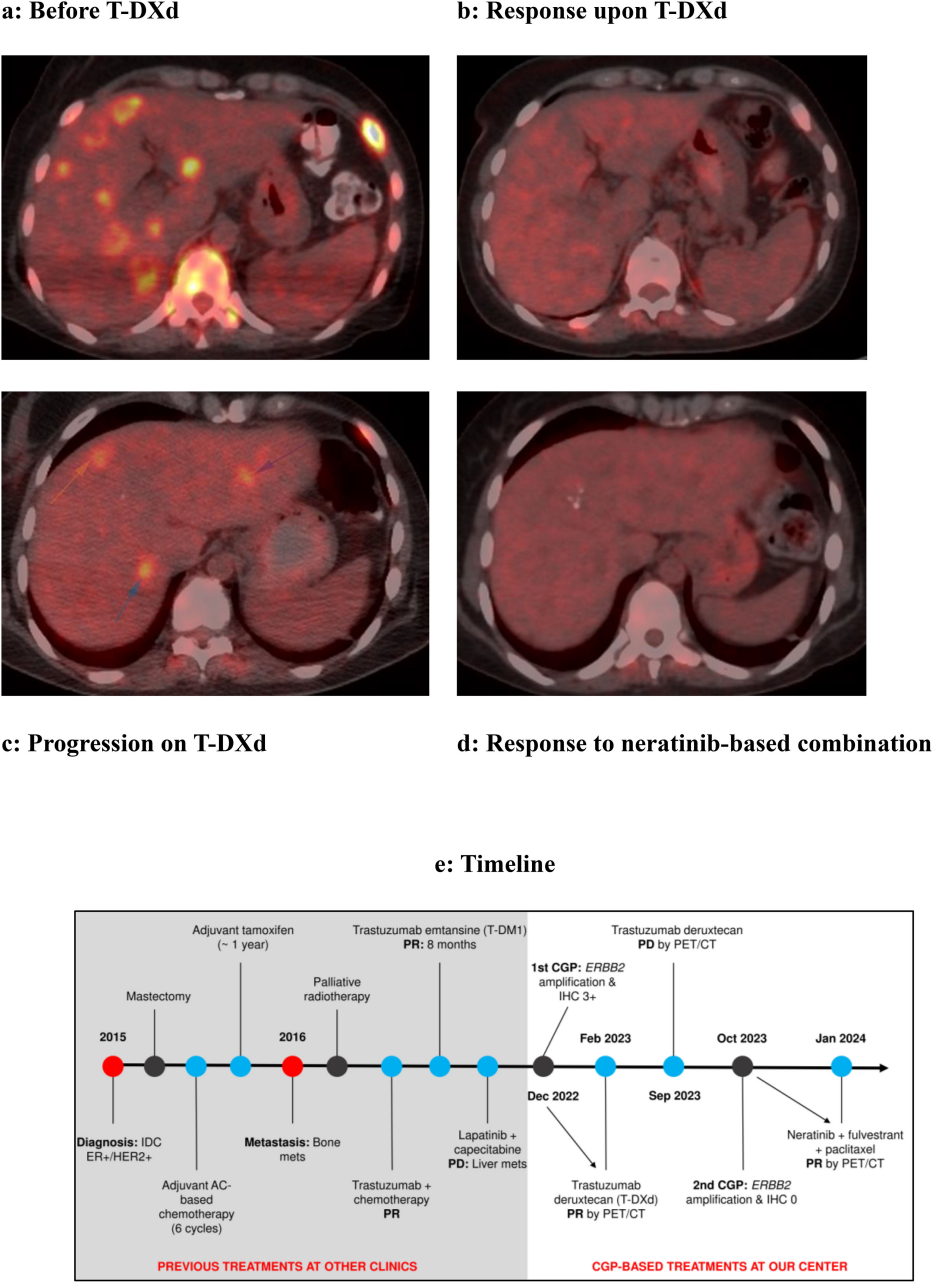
Near-complete responses in an HR+/*HER2-*amplified metastatic breast cancer patient, initially with trastuzumab deruxtecan (HER2 IHC–positive) and then with a neratinib-based combination (IHC-negative). (A) The December 2022 PET result showed multiple lesions with FDG uptake in the liver parenchyma consistent with metastasis. (B) The PET scan in February 2023 after T-DXd treatment did not reveal any lesions with significant FDG uptake. (C) In the September 2023 PET result, liver parenchyma shows several lesions with FDG uptake in the right and left lobes, consistent with metastasis (arrows). (D) In the January 2024 PET examination, no lesions showing FDG uptake could be detected in these lobes. (E) Disease and treatment timeline.
